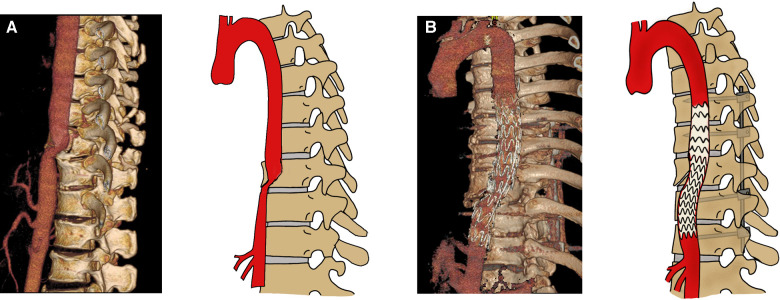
Computed tomography angiograms obtained before (A) and after (B) endovascular repair of the thoracic aortic injury and fixation of the spinal fractures.
